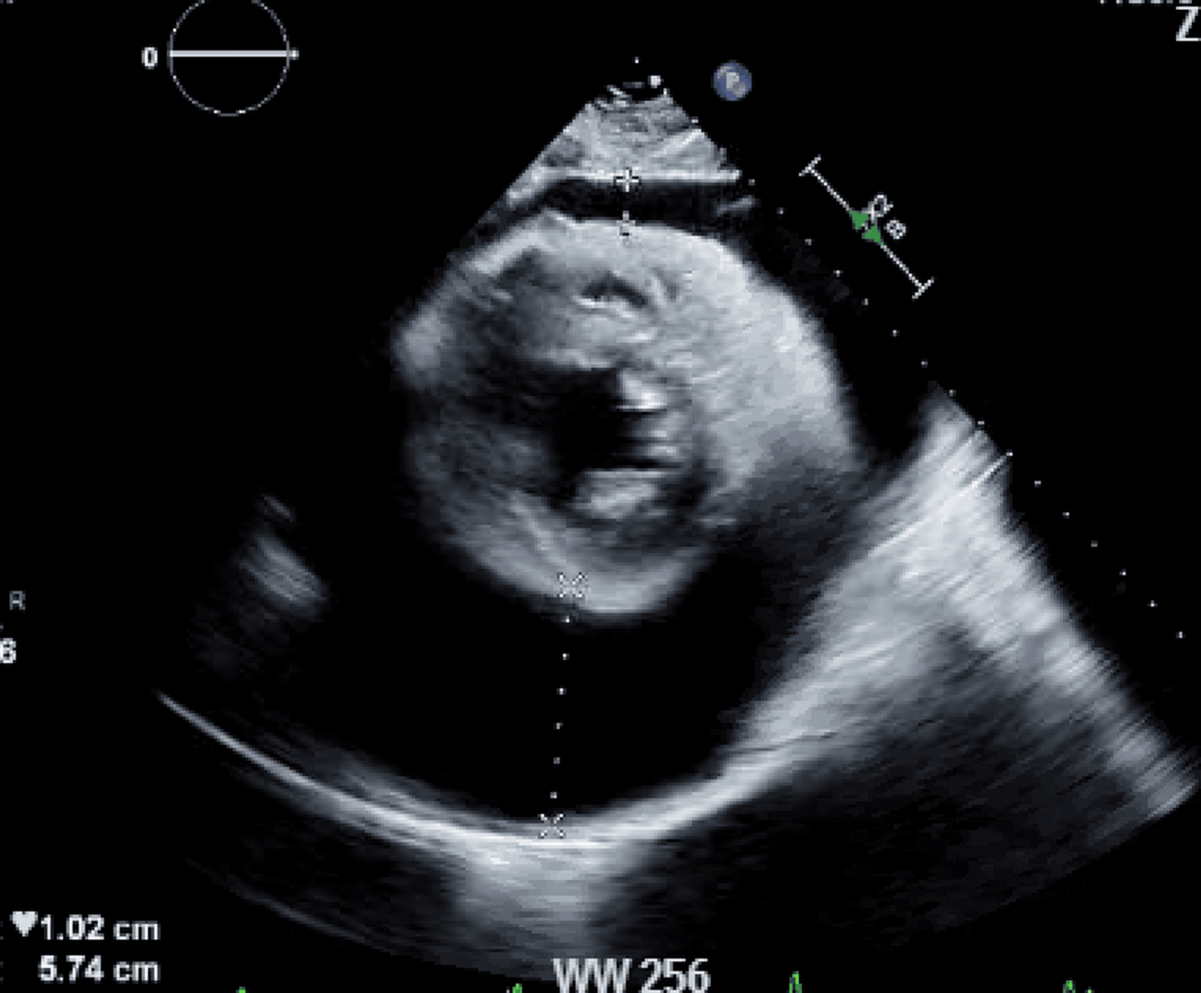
Transthoracic echocardiography showing circumferential anechoic space consistent with pericardial effusion.
